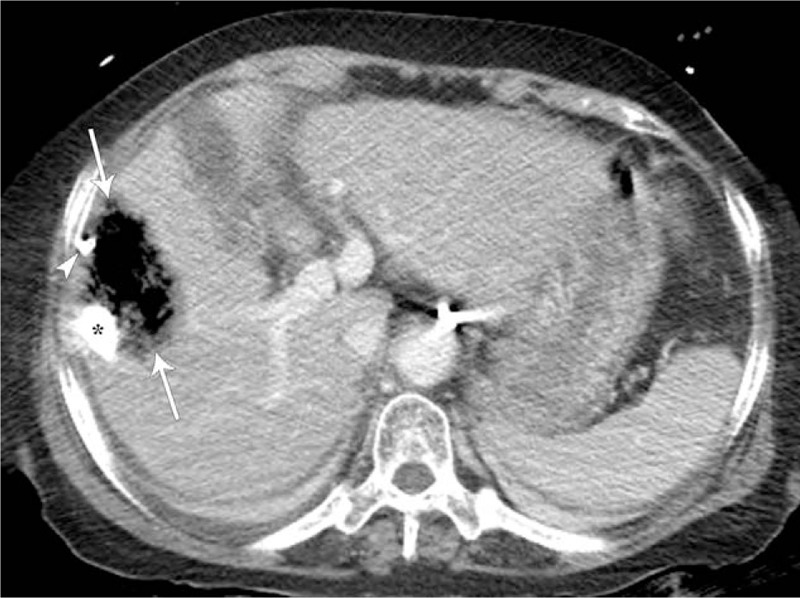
Three-week follow-up contrast-enhanced axial CT image after second TACE demonstrates a pigtail catheter (arrowhead) for percutaneous drainage of an aggravated air-filled abscess (arrows) around a compact lipiodolized lesion (asterisk) in the right hepatic lobe. CT = computed tomography, TACE = transarterial chemoembolization.
